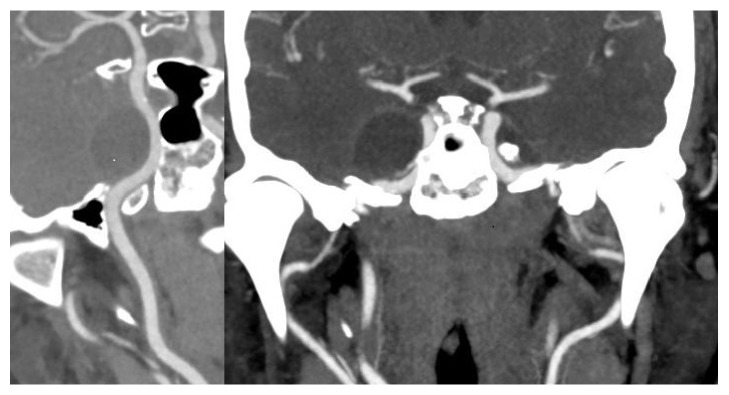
The CT angiography shows that the tumour is adjacent to the wall of the right internal carotid artery.
